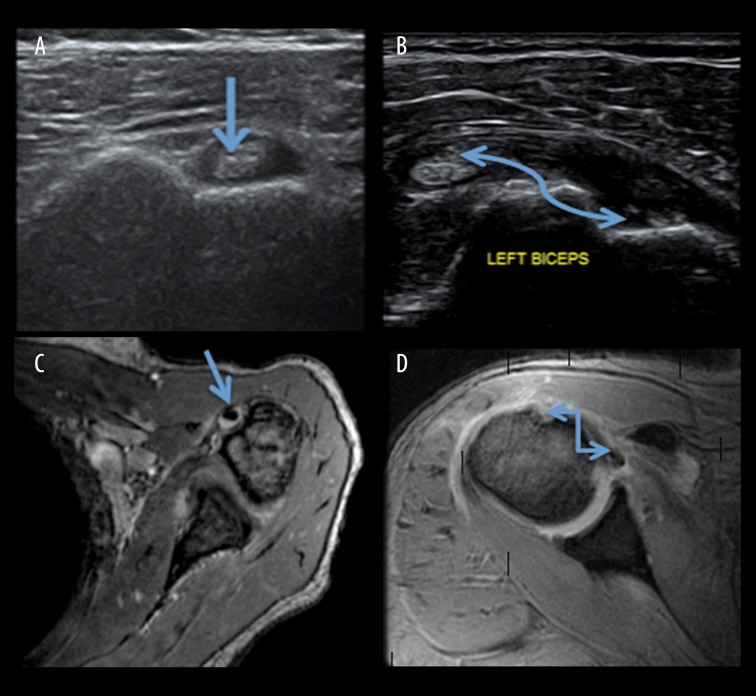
(A) USG image showing a normal biceps tendon in the bicipital groove (B) USG image showing non-visualization of the biceps tendon in the bicipital grove (C) MRI, T1W axial image depicting a normal biceps tendon in the bicipital groove (D) MRI, T2W axial image depicting non-visualization of the biceps tendon in the bicipital grove (arrow marked).
